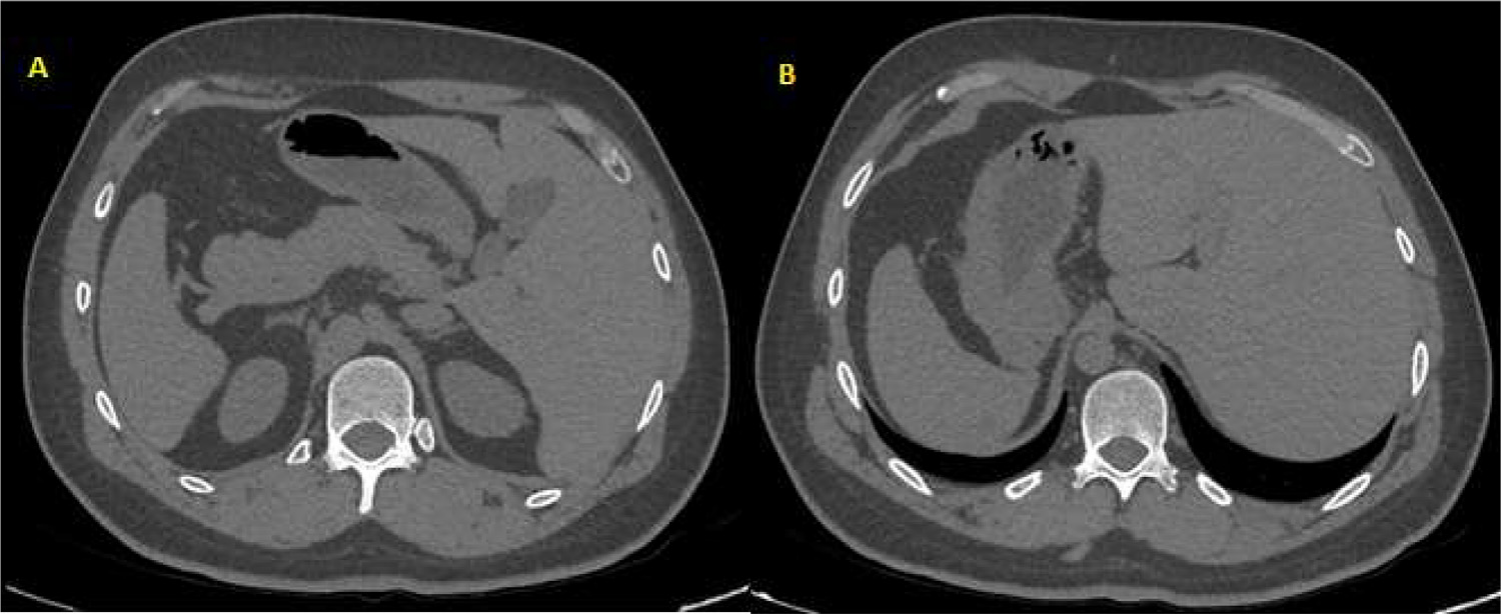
Axial CT image of the abdomen, without contrast medium at different levels. The anatomy of the abdomen was inverted: this is a condition of Situs Inversus Totalis (SIT). The stomach and spleen are on the left, and the bigger lobe of the liver is on the right.
